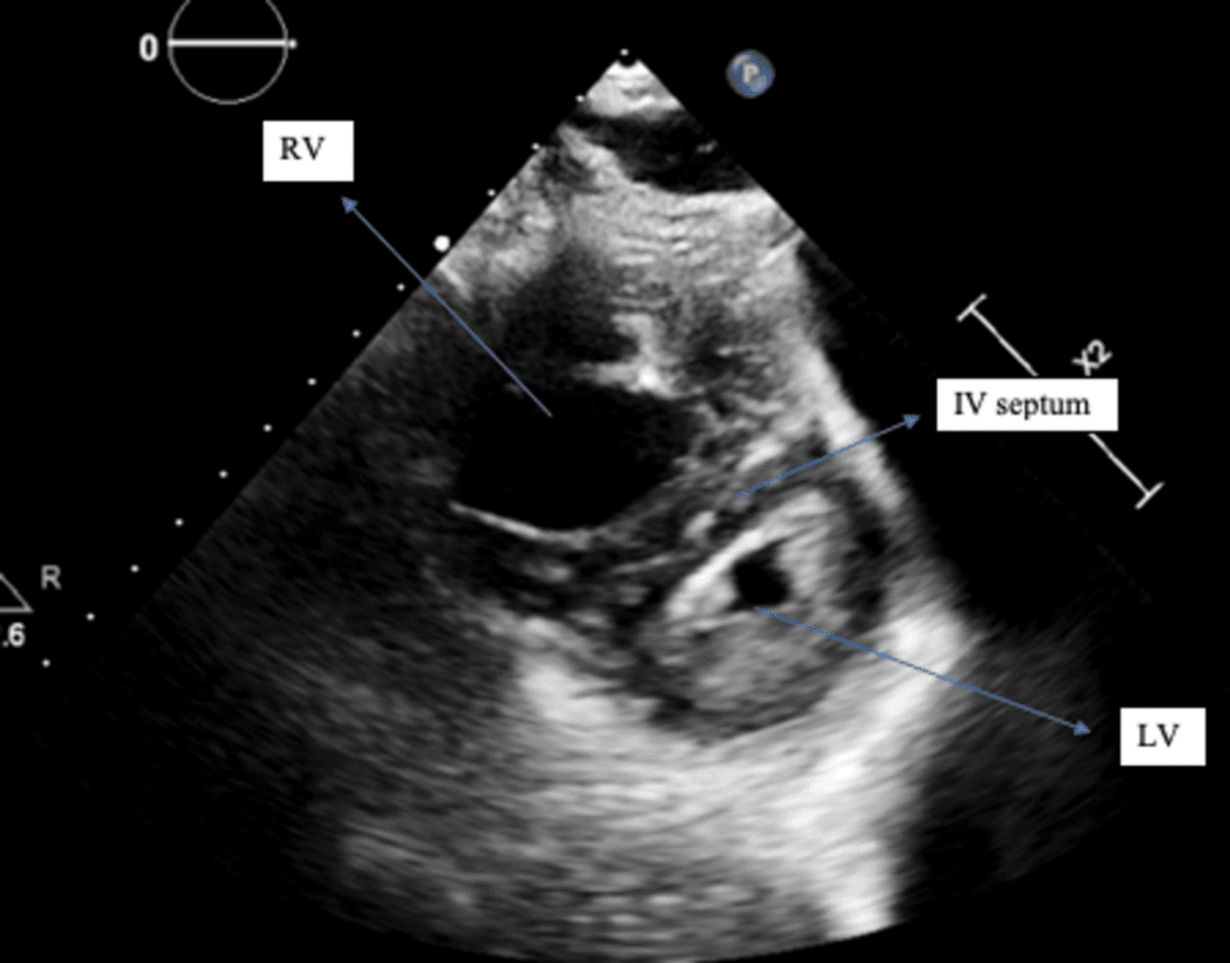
Transthoracic echocardiography (parasternal short axis view) showing severely dilated RV, small LV, and D-shaped interventricular septum. LV, left ventricle; RV, right ventricle
